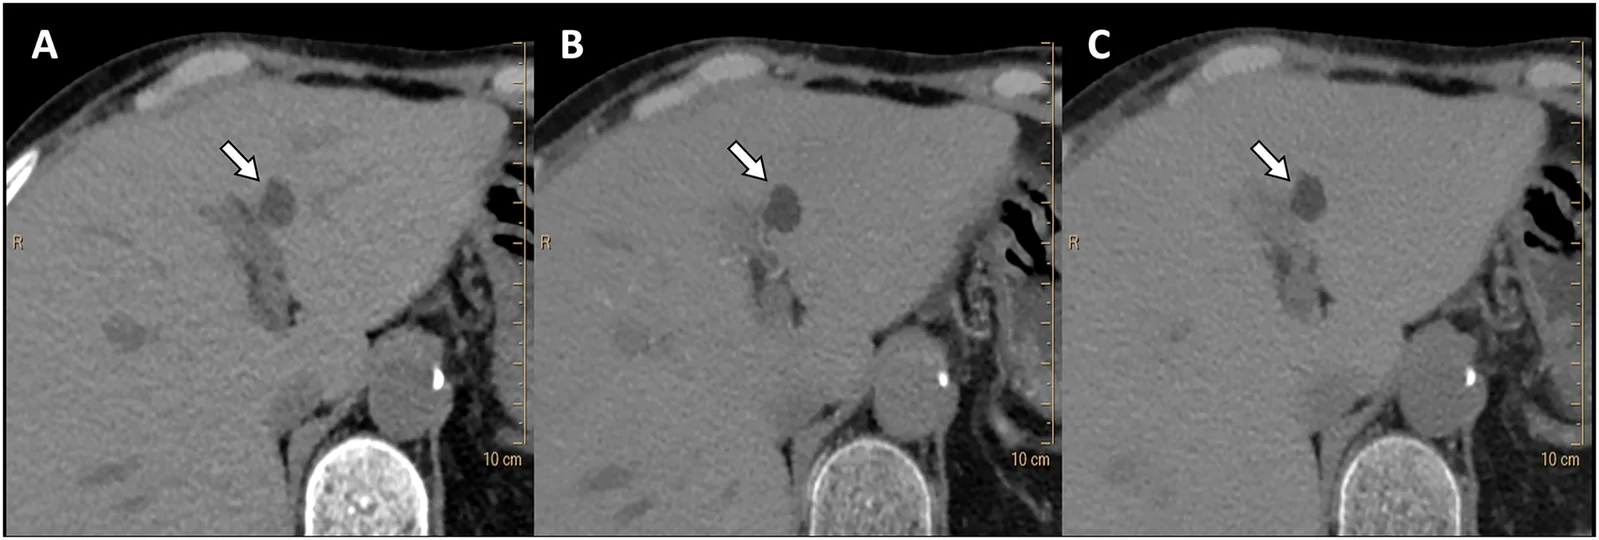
Axial CT images of a 46-year-old female patient with a hypodense liver lesion (arrow): A TNC image, B VNC_A image, and C VNC_P image (W 60 L 360)
